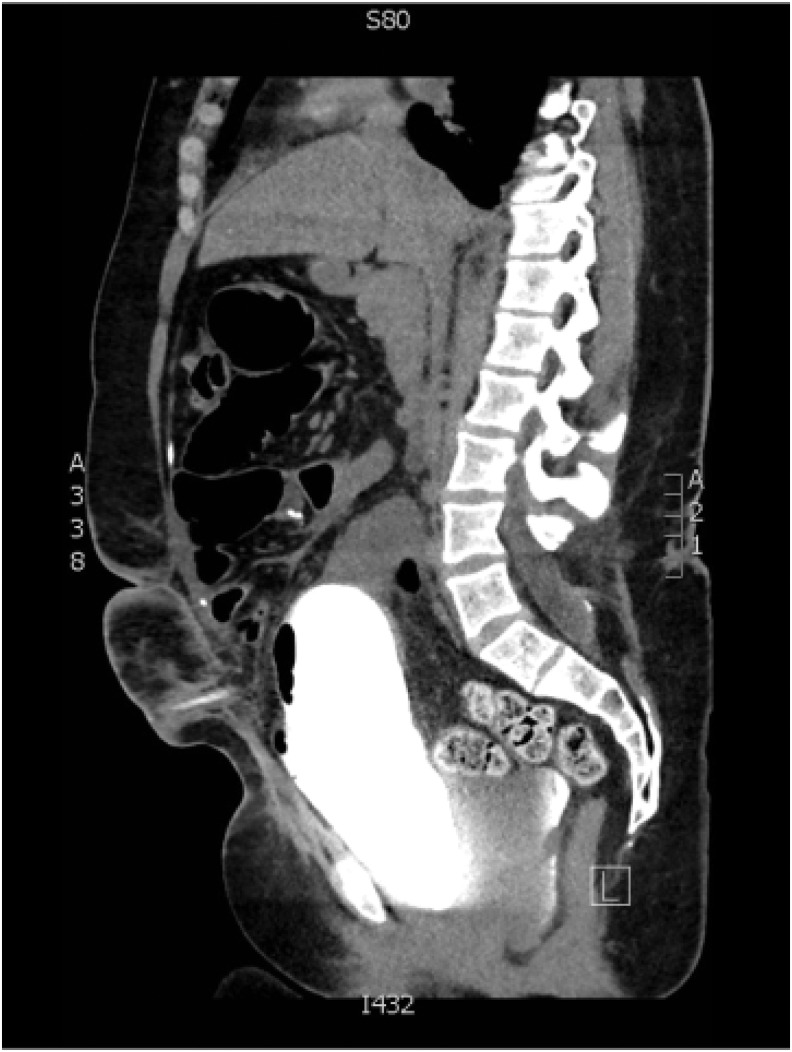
Sagittal imaging of CT cystogram showing posterior fluid collection and extravasation.
